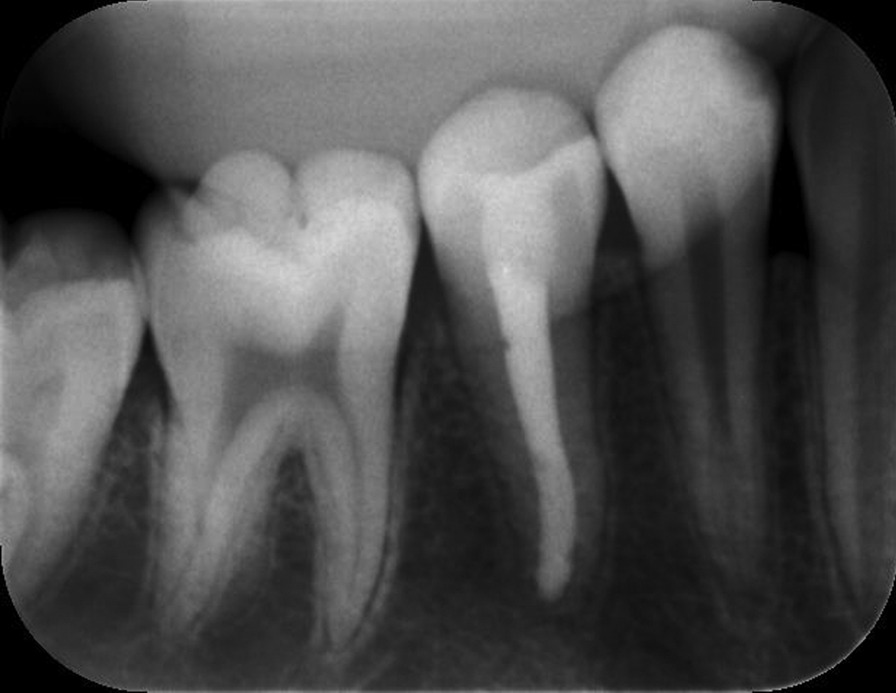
Radiography of the second premolar after 3 months of treatment
